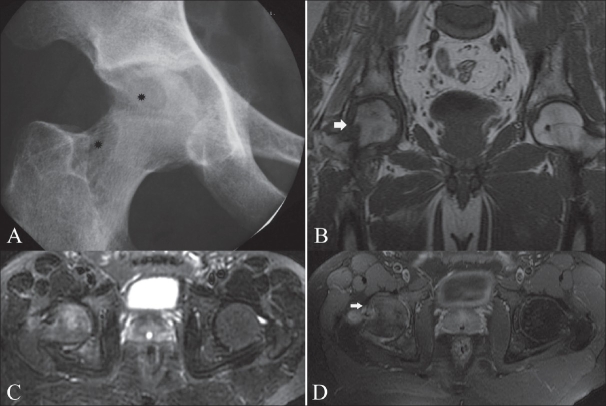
Tuberculous arthritis of the right hip. Plain radiograph (A) of the right hip demonstrates periarticular osteopenia, indistinct margins of the articular surfaces, and erosions of the femoral head and neck (asterisks). Coronal T1W MRI (B) shows a large erosion in the femoral neck (white arrow) and hypointense bone marrow edema within the acetabulum and lateral aspect of the femoral head. Axial fat-suppressed T2W MRI (C) shows hyperintense bone marrow edema in the right femoral head and acetabulum. Axial fat-suppressed T1W MRI (D) after gadolinium contrast administration shows peripheral enhancement of the large erosion in the femoral neck (white arrow). Note also some enhancement of the bone marrow of the femoral head and acetabulum as well as of the joint space
